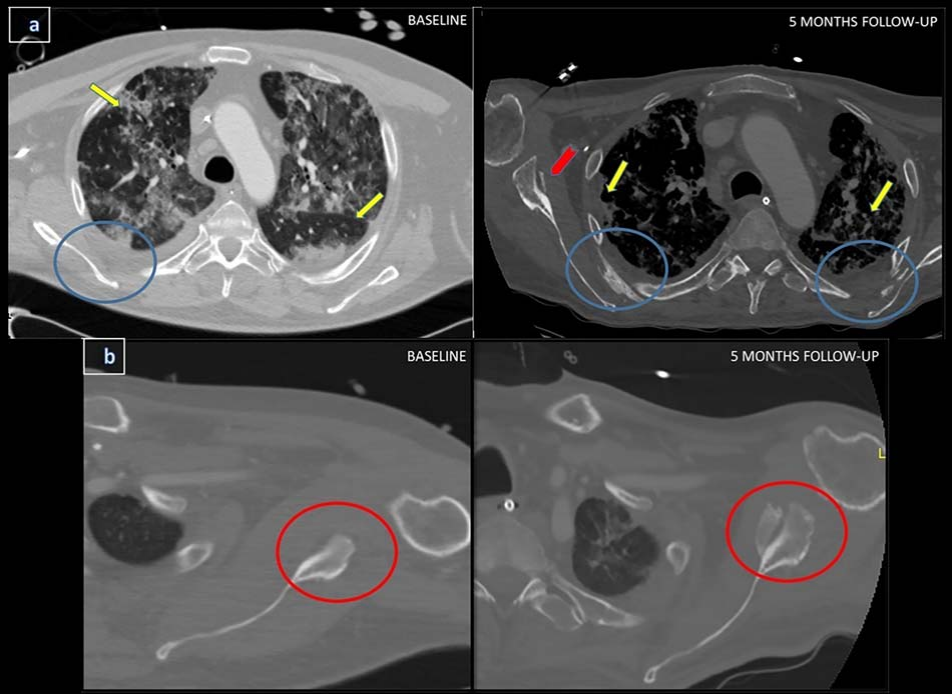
(a) Baseline axial thoracic-CECT images at aortic arch level and at 5 months follow-up axial thoracic-CECT images at the same level. Yellow arrows indicate the pulmonary findings related to COVID-19 pneumonia, represented mainly by bilateral ground glass opacities and peripheral lung consolidations. At the posterior chest wall region in baseline CT (left blue circles), we can confirm the absence of hyperdensity areas whereas in the follow-up CT, it clearly displays hyperdense linear images at the perimuscular soft tissue adjacent to the subscapular and intercostal muscles compatible with HO (right blue circles). In addition, at 5 months follow-up CT we can see a hyperdense linear image located in the perimuscular area adjacent to the right teres major muscle (red arrow). (b) Axial thoracic-CECT images at the left glenohumeral joint level and at 5 months follow-up axial thoracic-CECT images at the same level. Baseline CT shows the region adjacent to the teres major muscle (left red circle) without any involvement and in the control CT, it clearly shows hyperdense large nodular images of the perimuscular soft tissue adjacent to the teres major muscle (right red circle).
